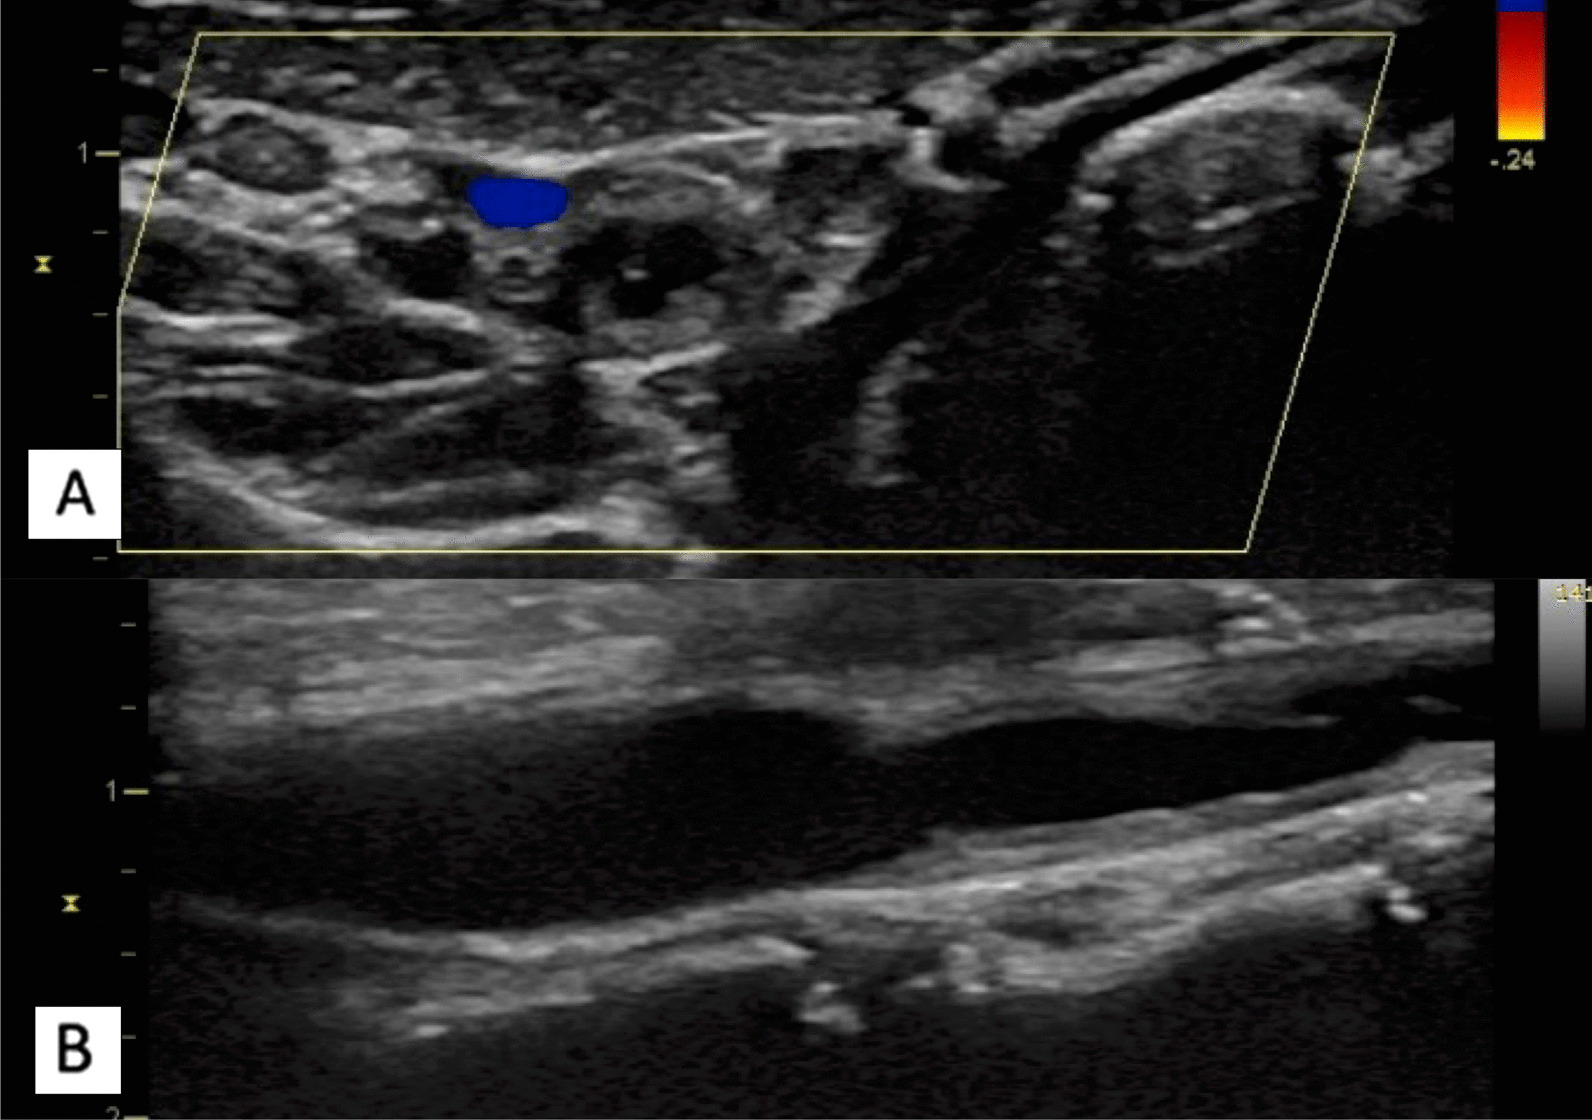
Carotid Doppler ultrasonography. Carotid ultrasonography showed a typical noncalcified homogenous lesion of Takayasu arteritis, causing an irregular vessel wall in the perpendicular (a) and longitudinal (b) views
